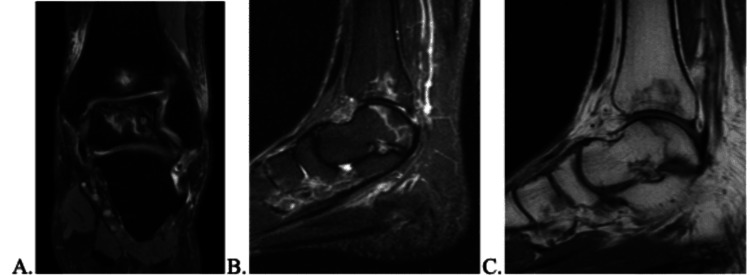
MRI from September 14, 2022, with no progression but continuation of AVN. AVN: avascular necrosis
